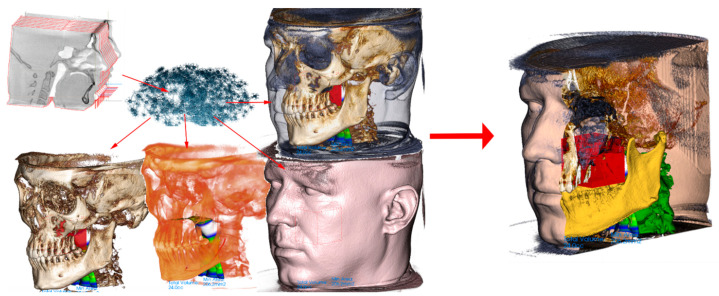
The examples of the segmentation process on the CBCT data based on defining ranges for particular tissues thus defining 3D structures such as airway, nerve canal, face surface or bone structures.
